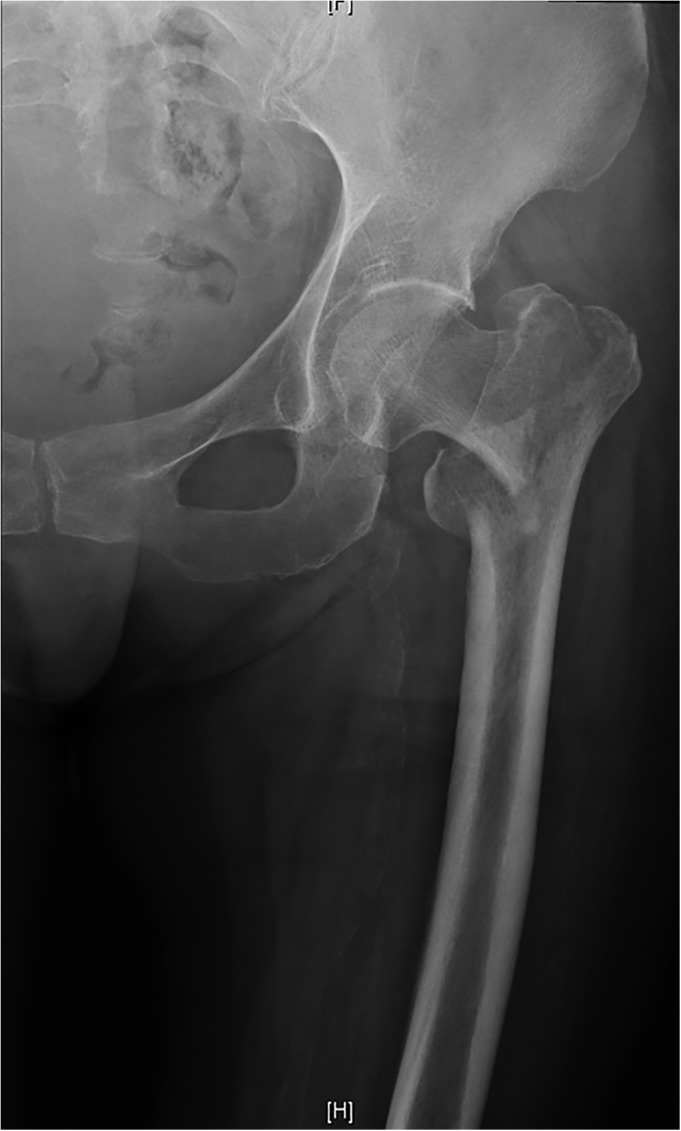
Anteroposterior radiograph showed an unstable intertrochanteric fracture.
